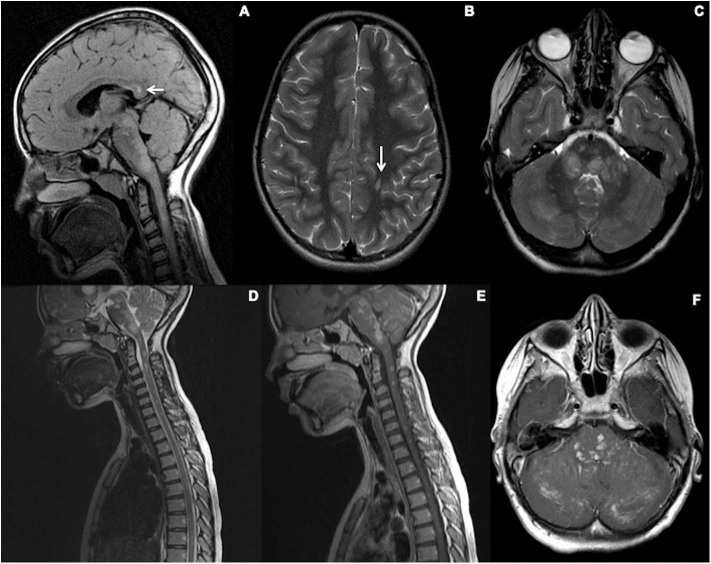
Top row (A–C): Initial imaging at age 12. (A) Sagittal T2 FLAIR-weighted imaging through the brain demonstrates evidence of signal hyperintensity within the calloso-septal interface, callosal splenium (white arrow), dorsal brainstem, and cervical spine. (B,C) Axial T2-weighted sequences of the brain show a juxtacortical lesion in the left perirolandic region (arrow) and ill-defined areas of abnormal signal in the pons and cerebellar hemispheres bilaterally (R>L).Bottom row (D–F)—Initial imaging at age 12. (A,B) Sagittal T2 and post-contrast T1-weighted sequences show the extent of signal abnormality within the brainstem and spinal cord. All the T2 hyperintense parenchymal lesions show enhancement. F-Axial post-contrast T1 Weighted sequence through the posterior fossa shows enhancement corresponding to the T2 hyperintense areas of abnormal signal in the pons and cerebellar hemispheres bilaterally (R>L). In addition, there is folial enhancement suggesting pial involvement.
